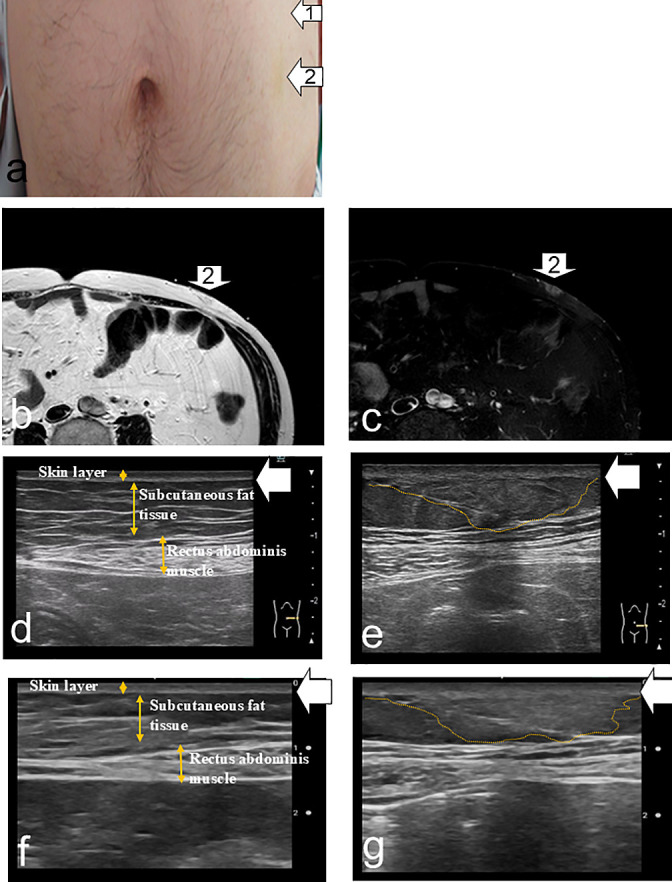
No subcutaneous induration or mass detected by visual inspection and palpation (Patient 3) a: Site with a low frequency of insulin injections (white arrow 1). Insulin injection site (white arrow 2). b: T2-weighted image of the insulin injection site (white arrow 2) showing low signal intensity in the region indicated by the arrow compared with the surrounding subcutaneous fat tissue. c: Fat-suppressed T2-weighted image of the insulin injection site (white arrow 2) demonstrating a hyperintense signal in the region indicated, relative to the surrounding subcutaneous fat tissue. d: High-resolution ultrasound image of the upper left abdomen at a site with a low frequency of insulin injections (white arrow 1) showing a well-defined dermis–subcutaneous fat boundary. The dermis (white arrow) is clearly visualized, and the subcutaneous fat exhibits normal echogenicity. e: High-resolution ultrasound image of the insulin injection site (white arrow 2) showing an indistinct dermis–subcutaneous fat boundary (white arrow) and altered echogenicity of the subcutaneous fat compared with the surrounding tissue (yellow dotted line). f: Pocket-sized ultrasound image of the upper left abdomen at a site with a low frequency of insulin injections (white arrow 1) showing a well-defined dermis–subcutaneous fat boundary. The dermis (white arrow) is clearly visualized, and the subcutaneous fat demonstrates normal echogenicity. g: Pocket-sized ultrasound image of the insulin injection site (white arrow 2) showing an indistinct dermis–subcutaneous fat interface (white arrow) and altered echogenicity of the subcutaneous fat relative to the surrounding tissue (yellow dotted line).
